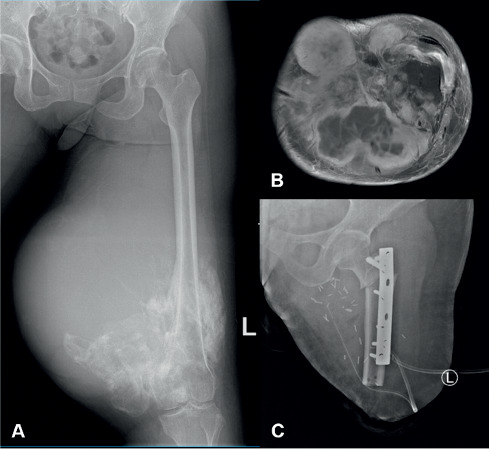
A 37-year-old female (case number 6) manifested local recurrence after misdiagnosed as osteochondroma and underwent multiple simple excisions. Preoperative radiograph of the left femur depicting an expansive juxtacortical bone tumour with malignant characteristics (a). An axial T2-weighted MR image demonstrating tumour extension to posterior and medial compartment of the femur, with displacement and invasion of major neurovascular bundles (b). Showing a dedifferentiated pattern on core biopsy, the patient finally underwent transfemoral amputation and tibial augmentation (c) at our center.
